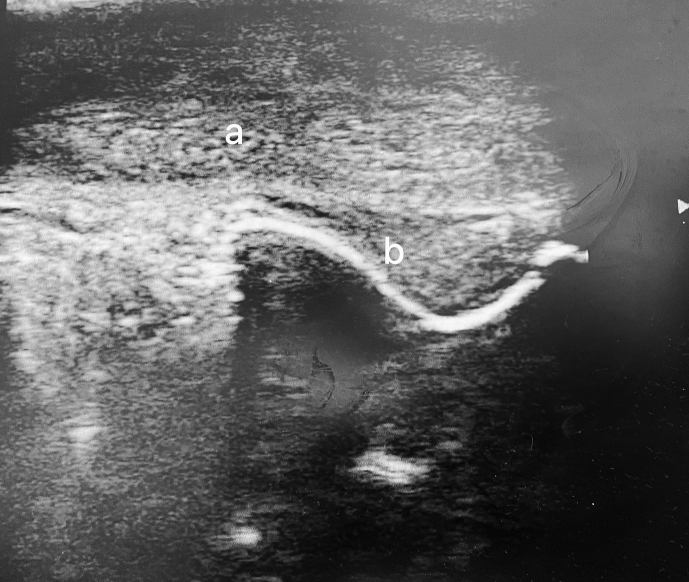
Penile ultrasound showing the corpus cavernosum (a) and the haematoma (b).
